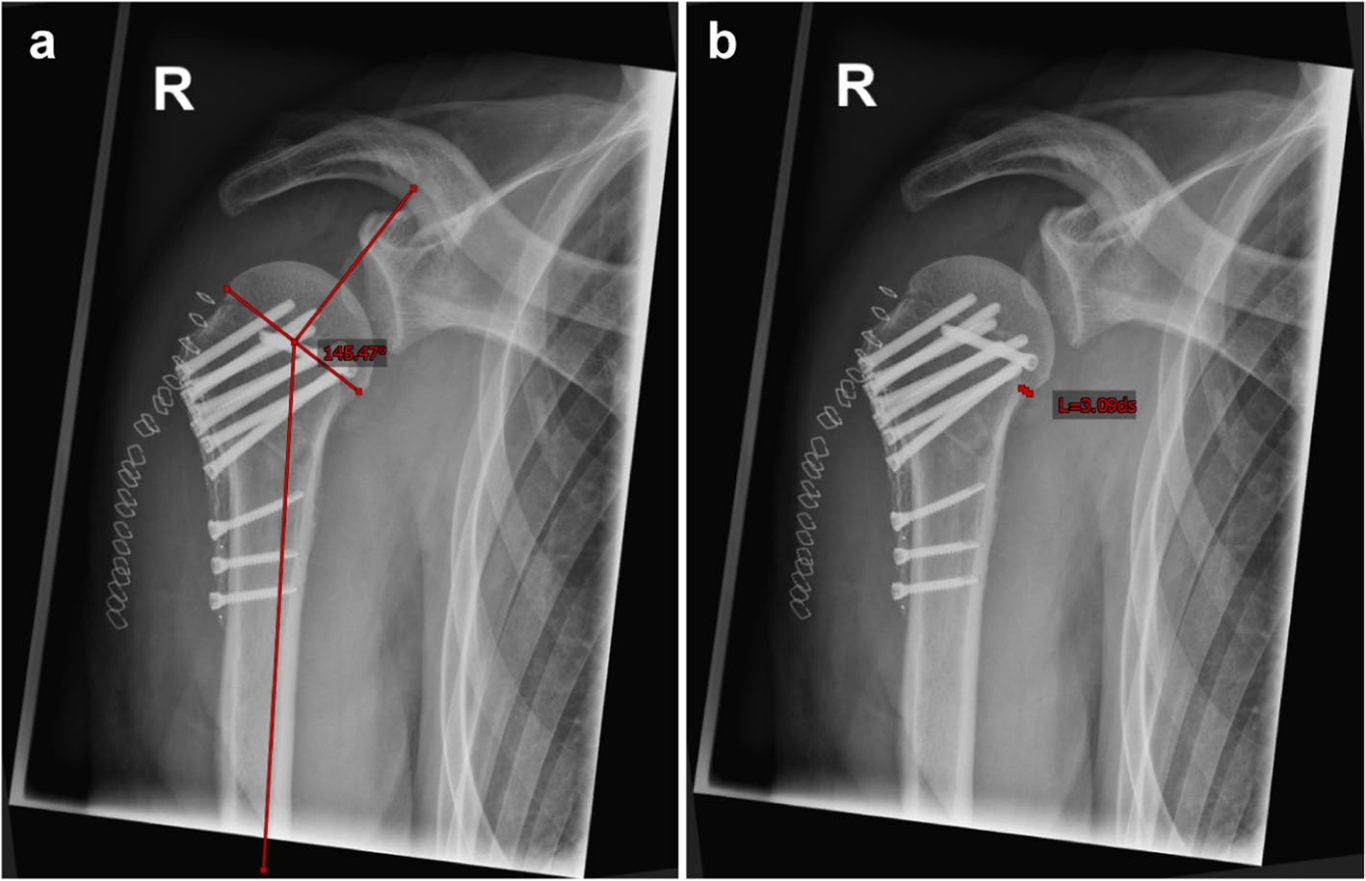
Radiological measurements. Postoperative radiological measurements: radiographs in ap view with a) neck shaft angulation, b) head shaft distance (ap = anteroposterior)
